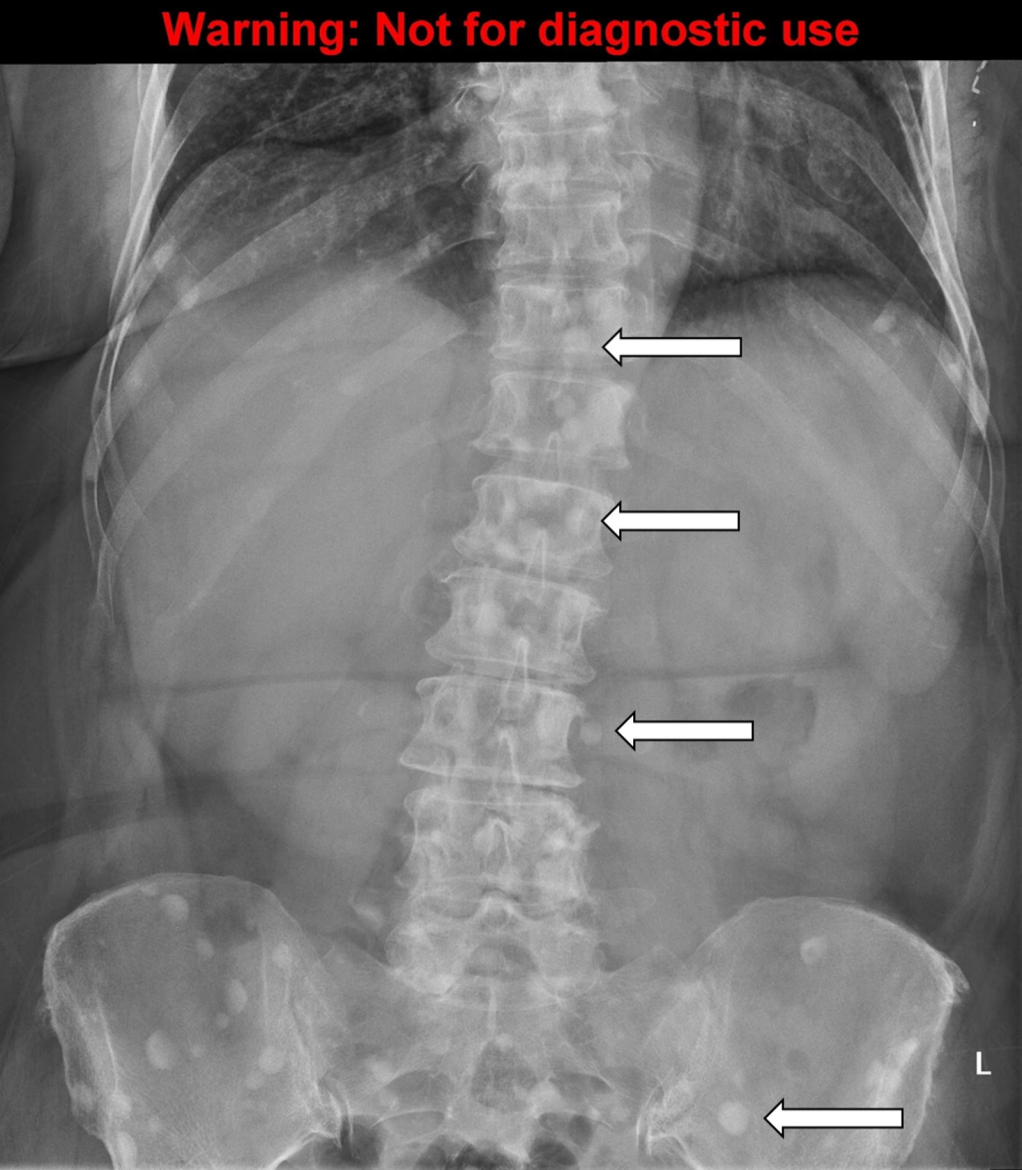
The abdominal X-ray demonstrating bone metastasis. Source: A Dixit
